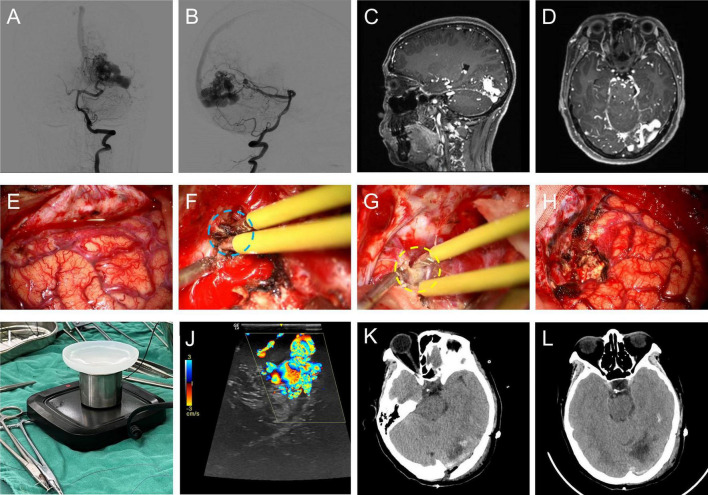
A typical case of brain AVM microsurgery using bone-wax coated bipolar electrocoagulation for hemostasis intraoperatively. Anterior (A) / Lateral (B) DSA image of the left vertebral artery; sagittal (C) / horizontal (D) MRA image of the brain AVM; (E) the exposed occipital cortex; intraoperative hemostasis using conventional bipolar electrocoagulation (F) / bone-wax coated bipolar electrocoagulation (G); (H) occipital cortex after resection of the brain AVM lesion; (I) intraoperative photograph of the bone-wax coated bipolar electrocoagulation application device; (J) intraoperative ultrasound exploration of the brain AVM lesion; CT image of the operated area on the first day of postoperative review (K) / on the fifth day of postoperative review (L).
